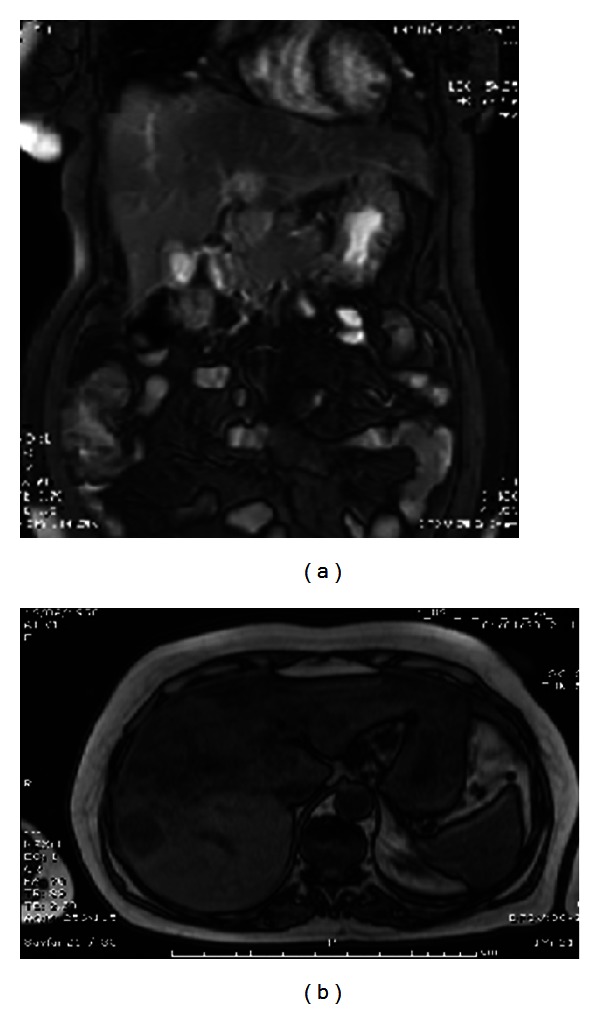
Coronal and axial images from control MRI.
